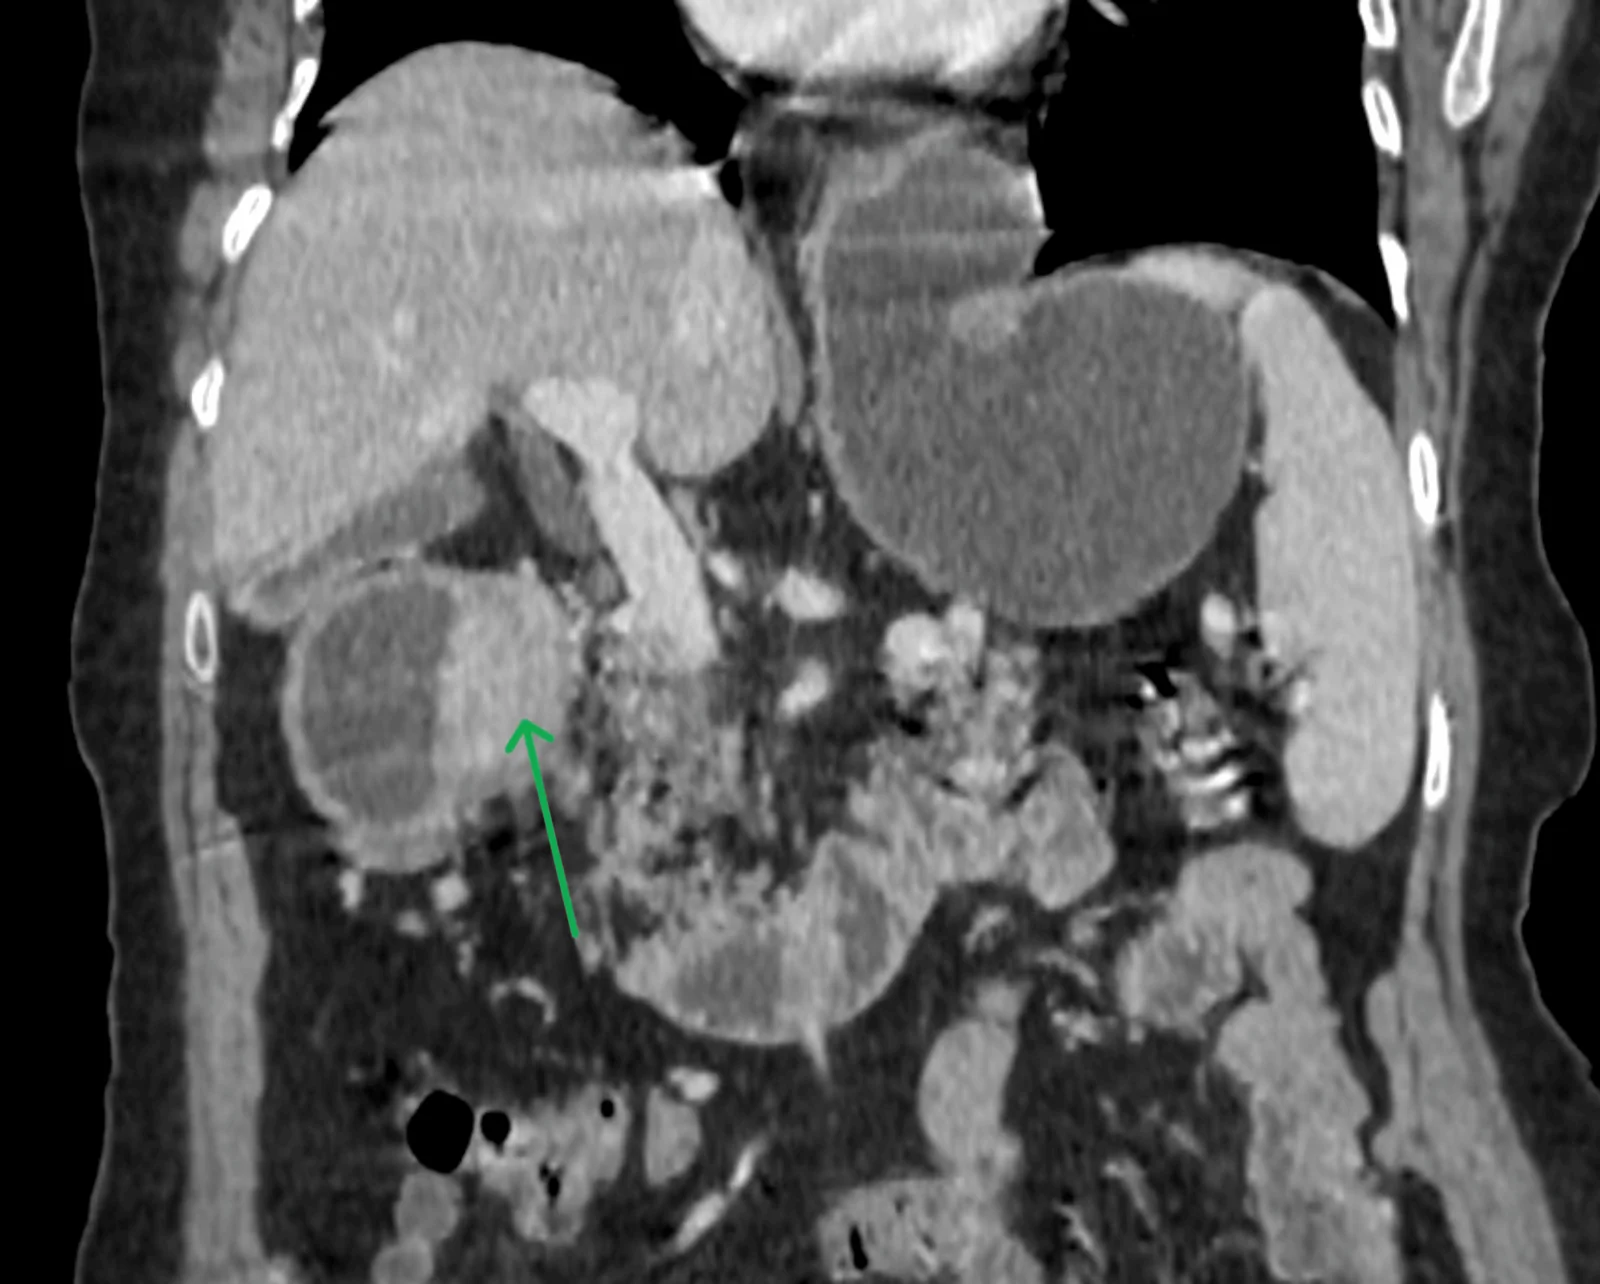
Contrast-enhanced abdominal computed tomography, coronal reconstruction, showing extension of the polypoid lesion toward the duodenal bulb (green arrow).
